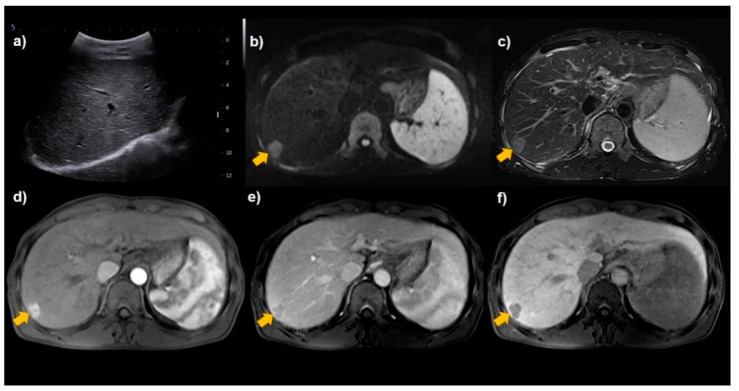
A 60-year-old male patient with chronic hepatitis B. (a) No focal hepatic lesion was found in surveillance ultrasonography due to poor sonic window. (b–f) Surveillance MRI detected a 2.0 cm nodule in segment VII (arrows). MRI shows diffusion restriction (b) and mild-to-moderate T2 hyperintensity (c) with arterial-phase hyperenhancement (d), portal venous washout (e), and hepatobiliary-phase hypointensity (f), suggesting HCC.
